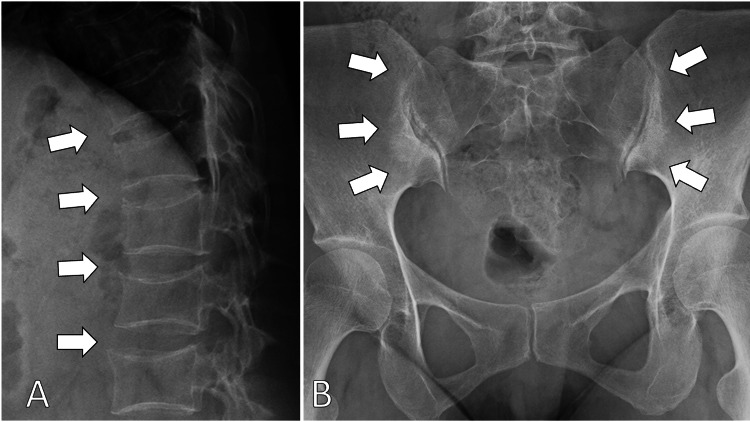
X-ray of the back (A) and pelvis (B) showing multiple bone spurs in the thoracic and lumbar vertebrae and the bilateral deformation of sacroiliac joints (white arrows)
